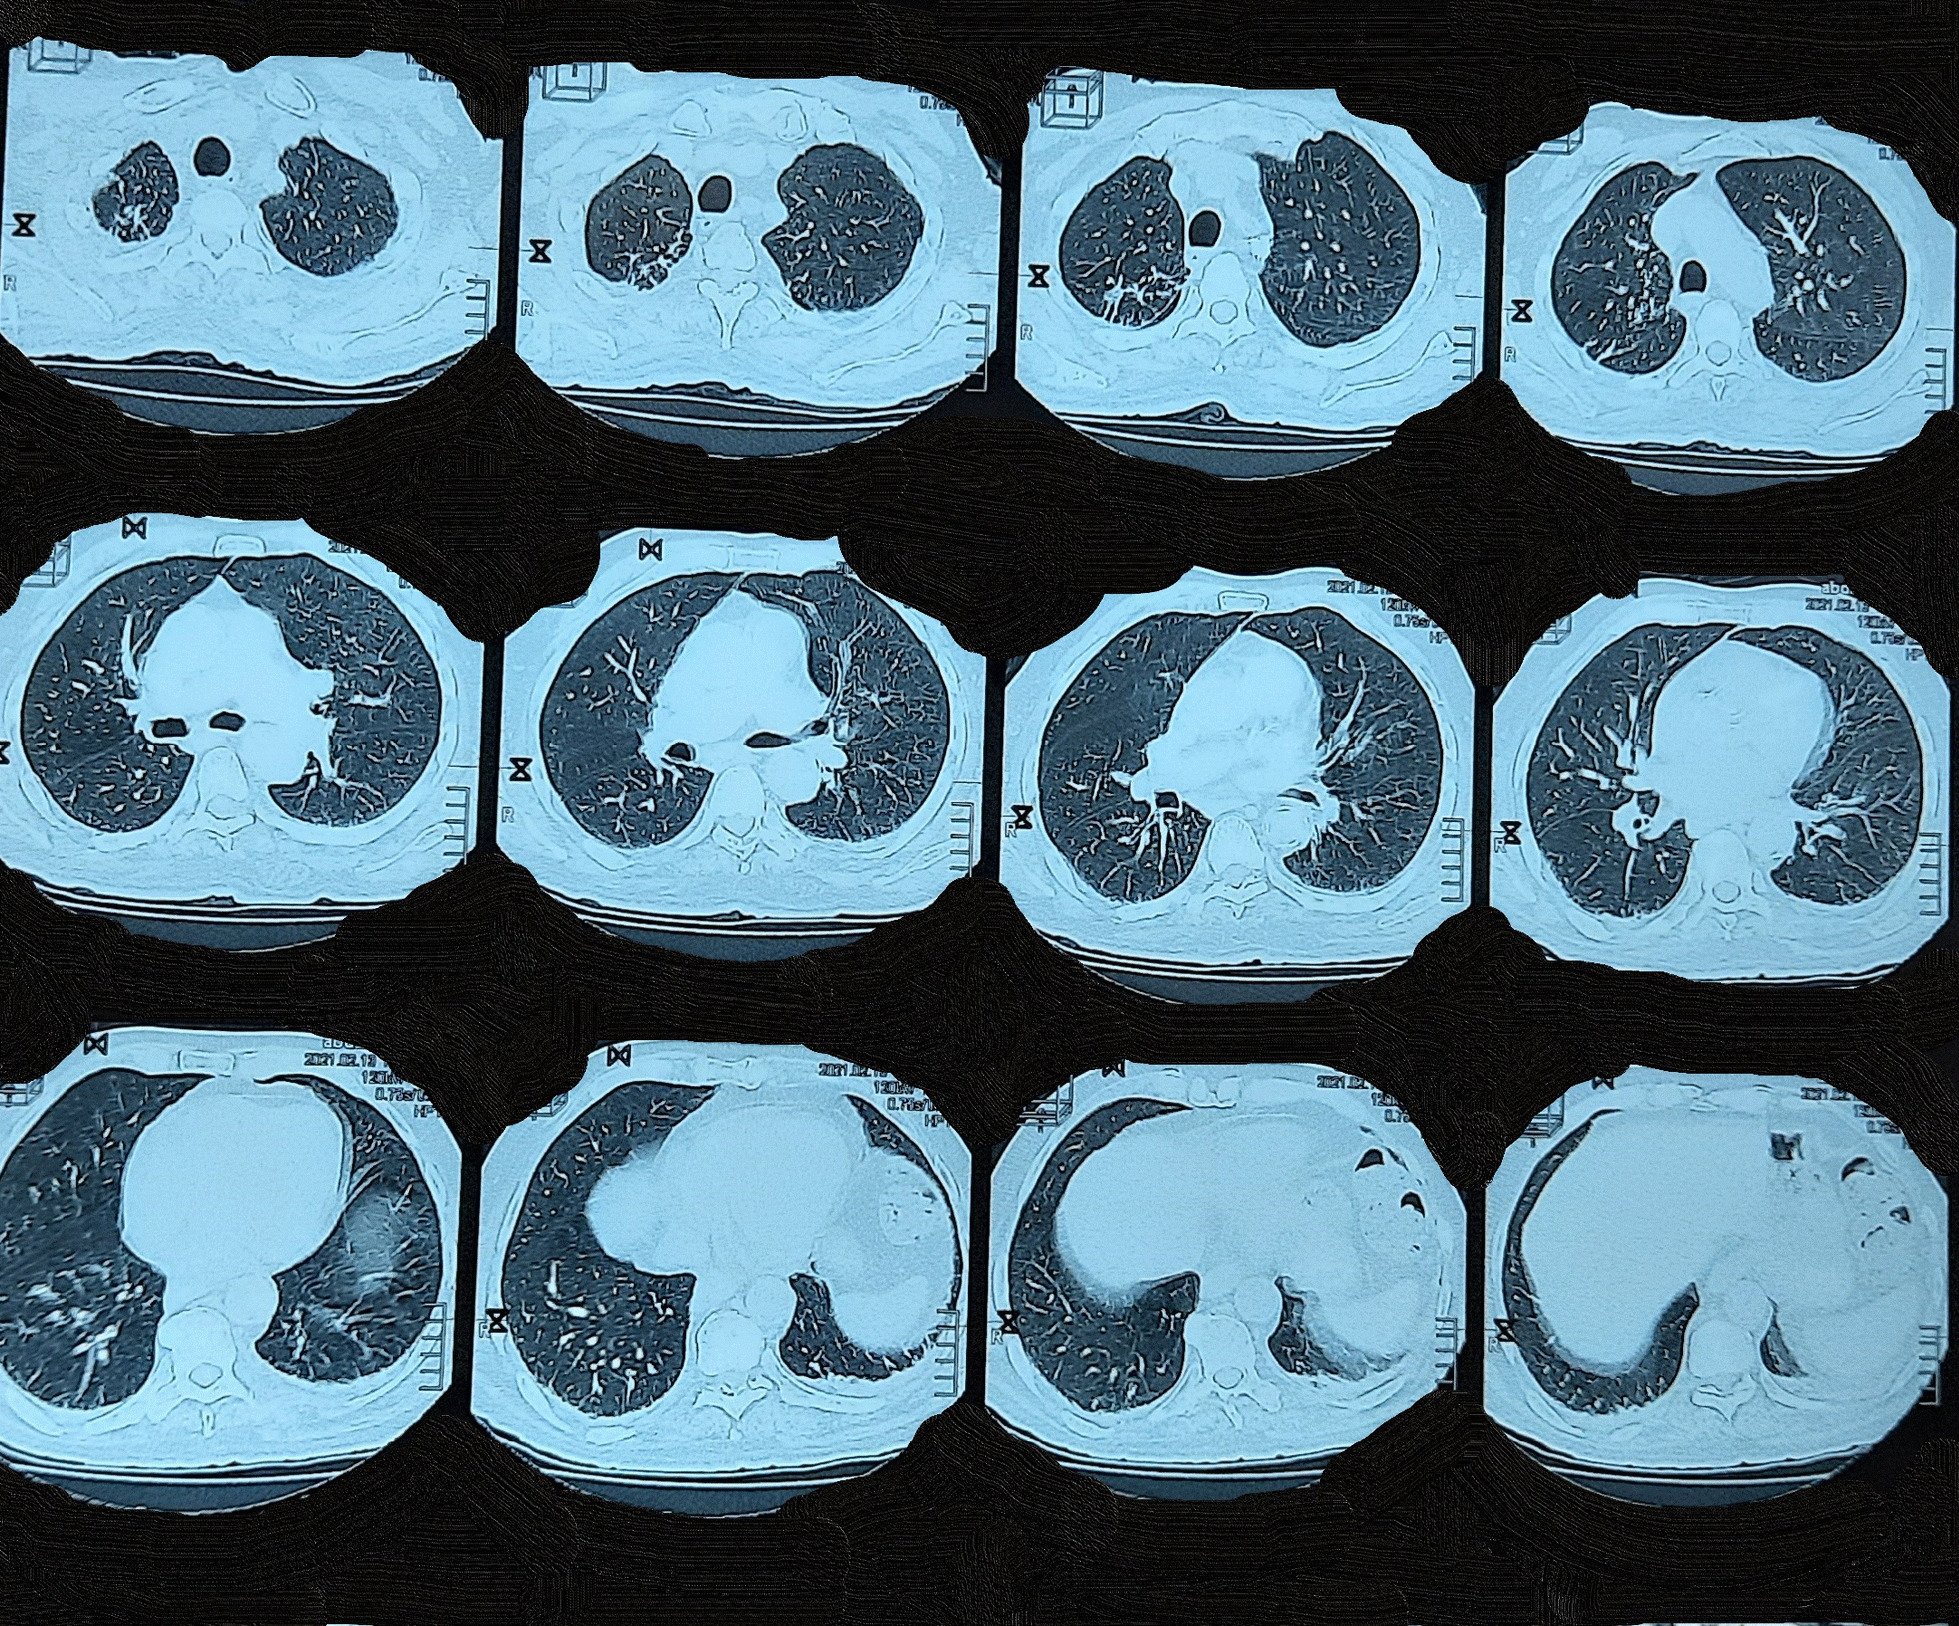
Chest computed tomography revealed only the presence of band-like opacities in the right upper lobe
